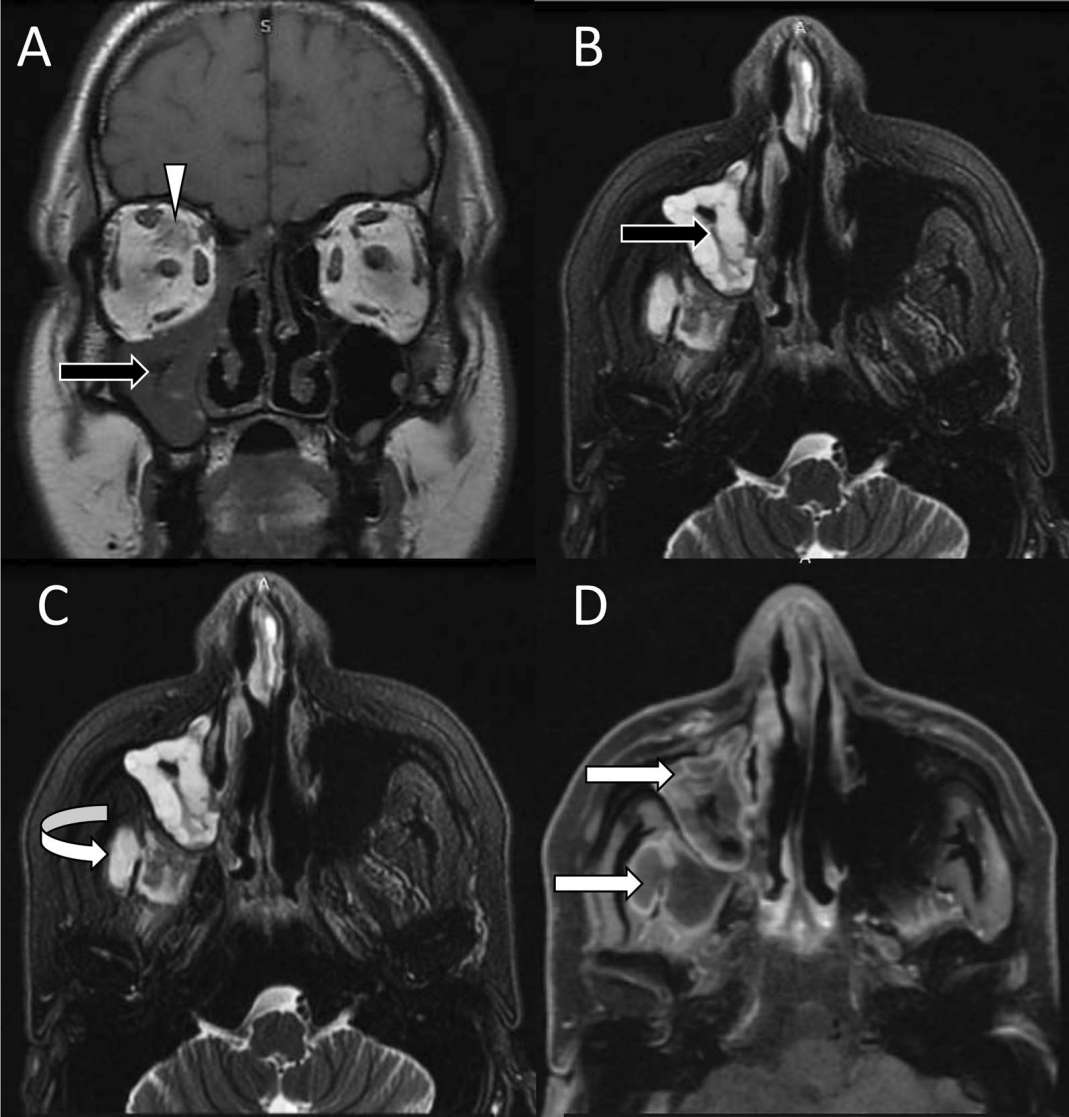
Sinus mucormycosis with extension to orbit and neck. Coronal T1-weighted (A) and axial T2-weighted fat-saturated (B, C) images show T1 hypointense and T2 hyperintense soft tissue (black arrows) in the right maxillary sinus extending posteriorly into retromaxillary neck space (curved white arrow). Contrast-enhanced axial T1-weighted fat-saturated image shows peripheral enhancement with central non-enhancing area (straight white arrows). Note the intraconal fat stranding in the image (A) suggesting orbital involvement (white arrowhead)
